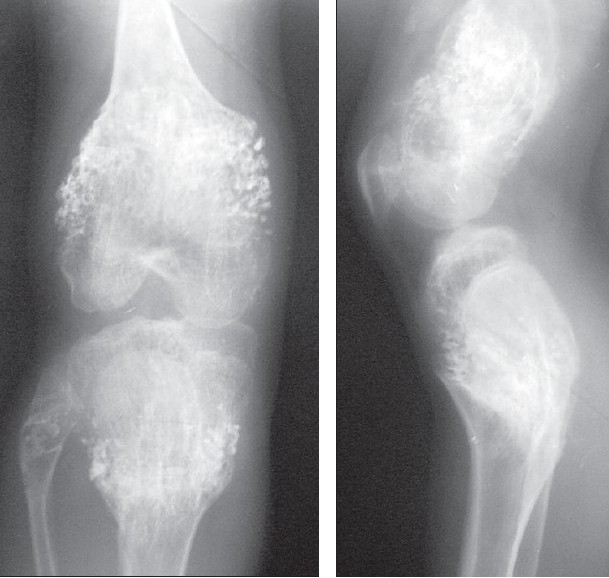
Anteroposterior and lateral radiographs showing multiple columnar cartilaginous masses of the right lower end of the femur and upper end of the tibia and fibula.
